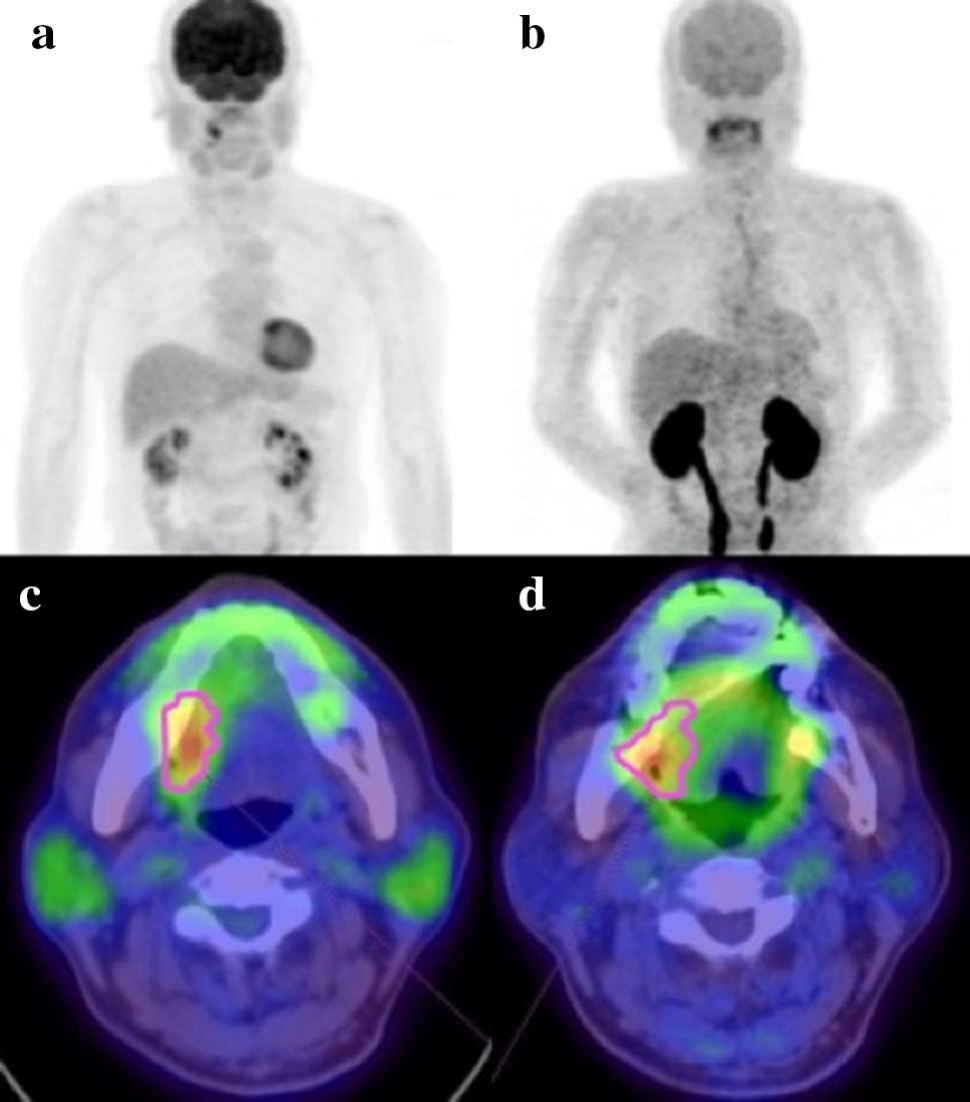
Maximum intensity projection imaging of a patient with a good outcome using a^18^F-FDG and b^18^F-FAMT and primary PET/CT imaging using c^18^F-FDG and d^18^F-FAMT
